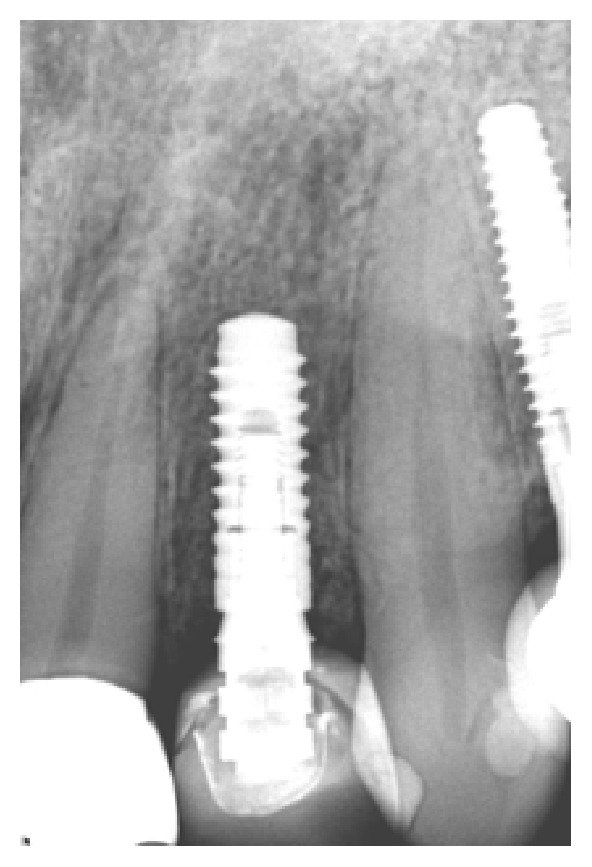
PA radiograph of provisional crown.
